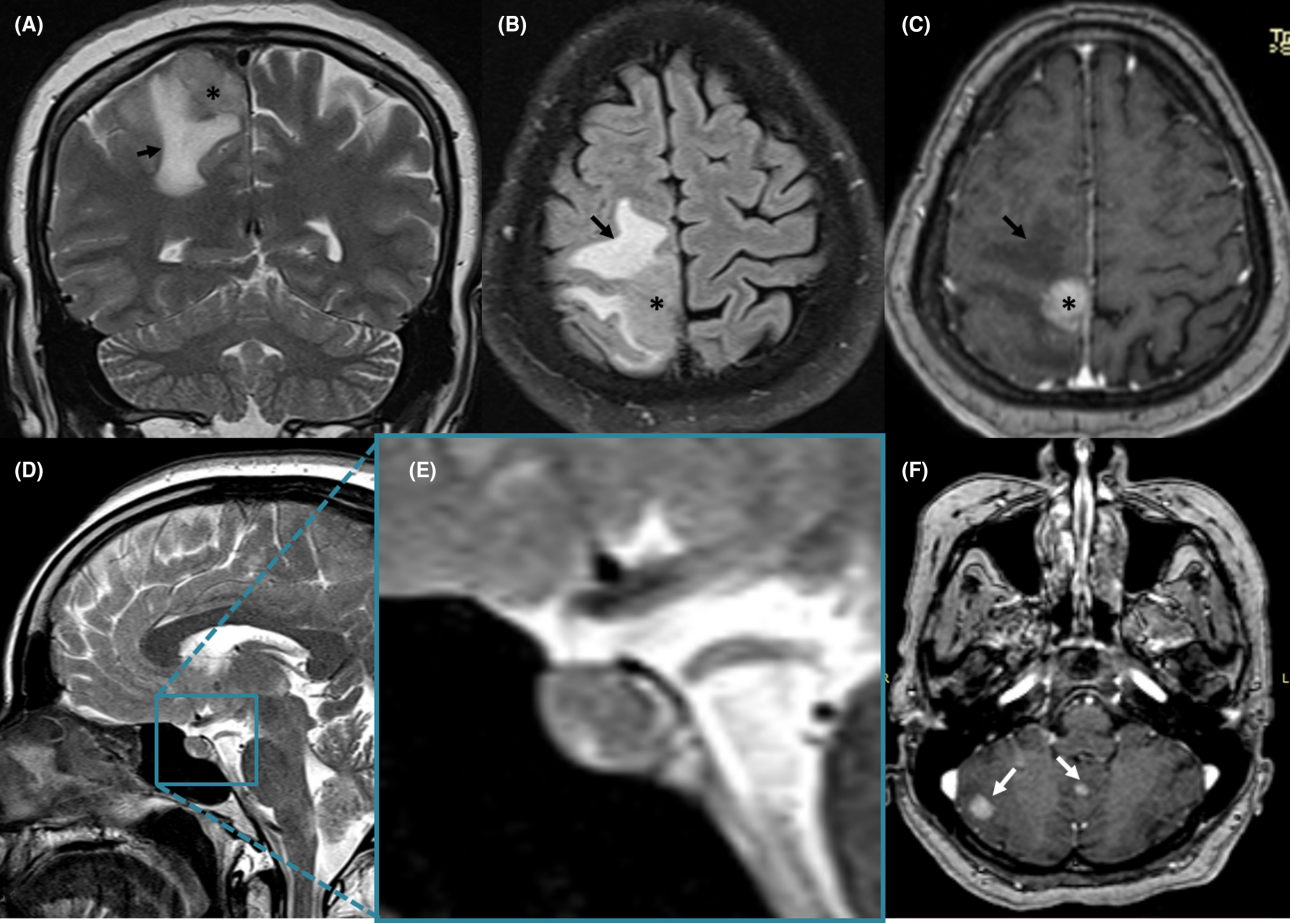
Parasagittal intra‐axial right rolandic mass lesion (white asterisk in “A–C”) presenting with T1, T2 (image A) and FLAIR (image B) isosignal and showing intense gadolinium enhancement (image C). Extensive fronto‐parietal vasogenic edema is seen around the lesion (black arrows in “A–C”). Images “D” and “E” show a normal hypophyse with no supra‐sellar lesion. Note the subtentorial metastatic lesions in the right cerebellum and the vermis (white arrows in “F”) presenting as round lesions with intense gadolinium enhancement
